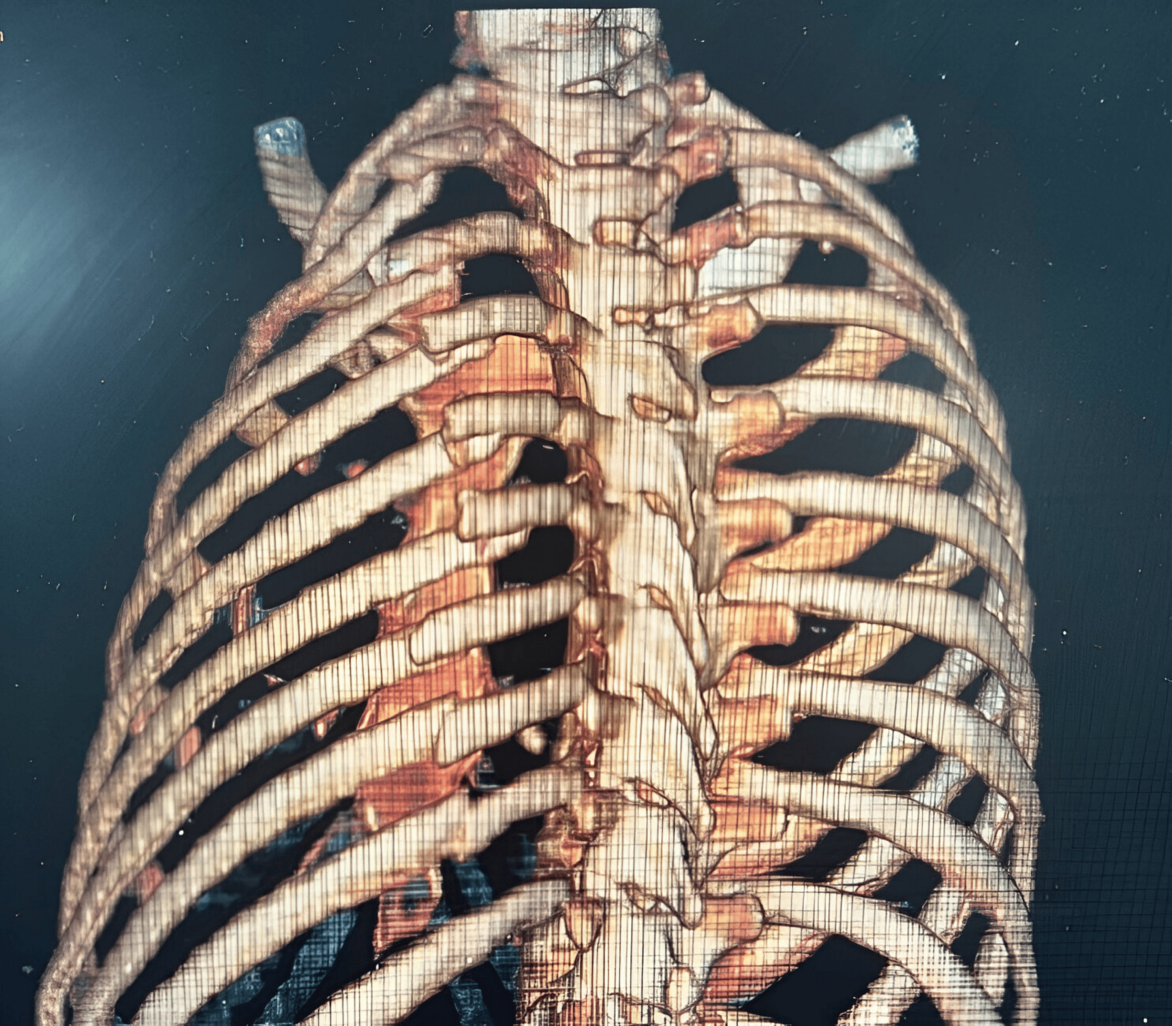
Chest computed tomography with 3D reconstruction showing evidence of displaced rib fractures from the second to the ninth rib of the left hemithorax in the postero-anterior view
